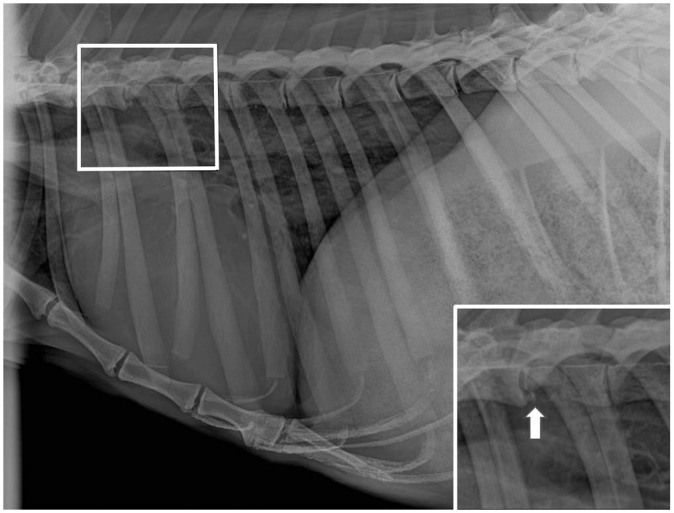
Lateral radiograph of the spine, thorax, and cranial abdomen of a Western grey kangaroo, with focal loss of opacity in T4 (in box). Inset: lytic T4 vertebral lesion highlighted (arrow).
